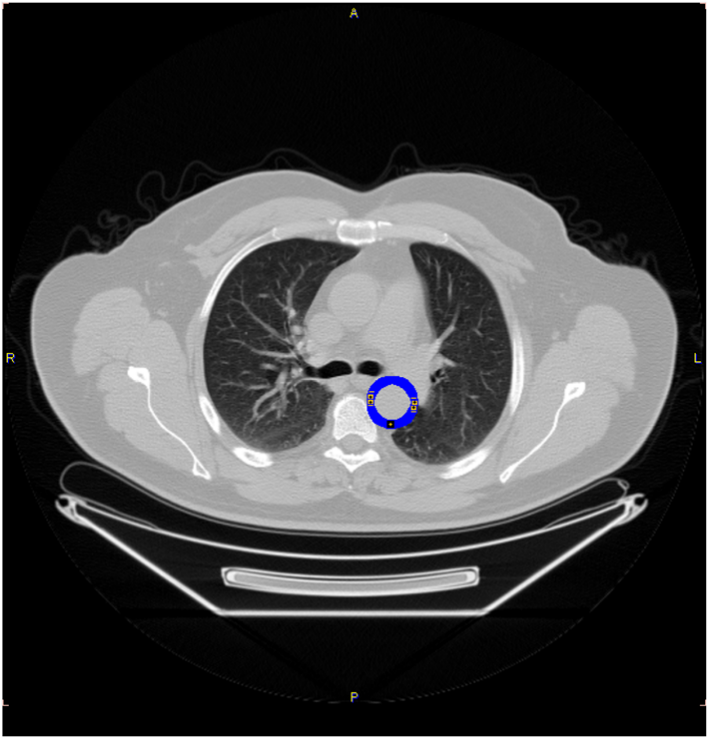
An example of the aorta VOI extraction on the image resulting from the fusion of CT and PET images of a diabetic patient. The blue circle represents the aorta VOI
